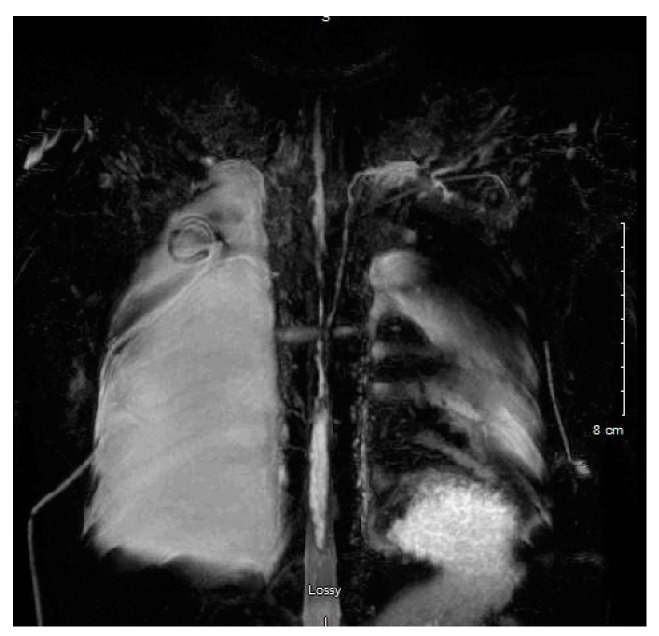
Magnetic resonance image (MRI) showed two separate lymphatic ducts draining the lymph from cisterna chyli to the right and left subclavian veins.
